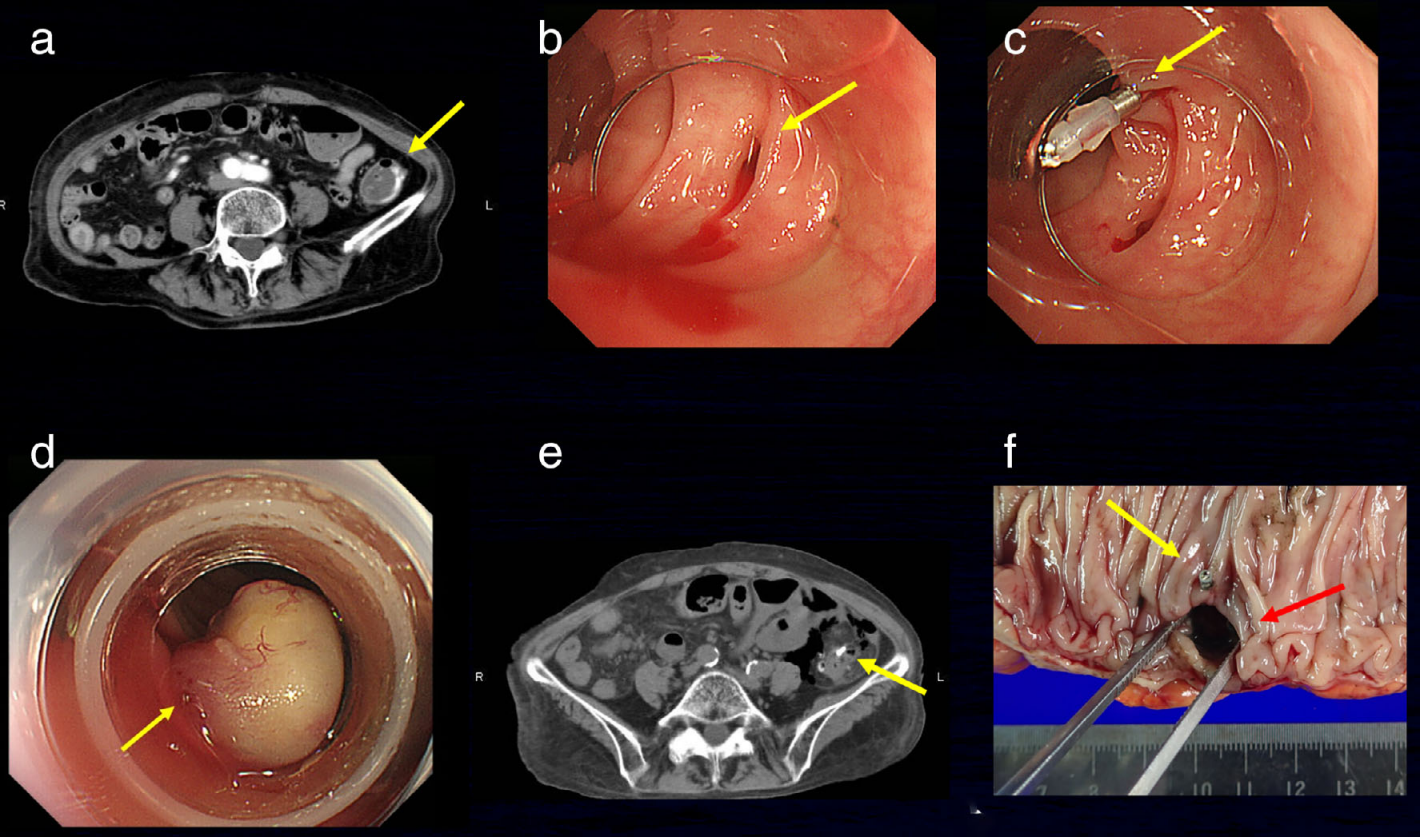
(a) Enhanced computed tomography demonstrating extravasation in the lower descending colon (arrow). (b) Bleeding is seen from the diverticulum (arrow). (c) The arrow shows the marking clip placed near the bleeding diverticulum. (d) The inverted diverticulum with exposed vessel (arrow) is ligated using endoscopic band ligation (EBL). (e) Emergency computed tomography demonstrating free air and mesenteric panniculitis in the descending colon around the marking clip (arrow). (f) A colonic wall defect is macroscopically visible at the EBL site (red arrow) near the marking clip (yellow arrow).
